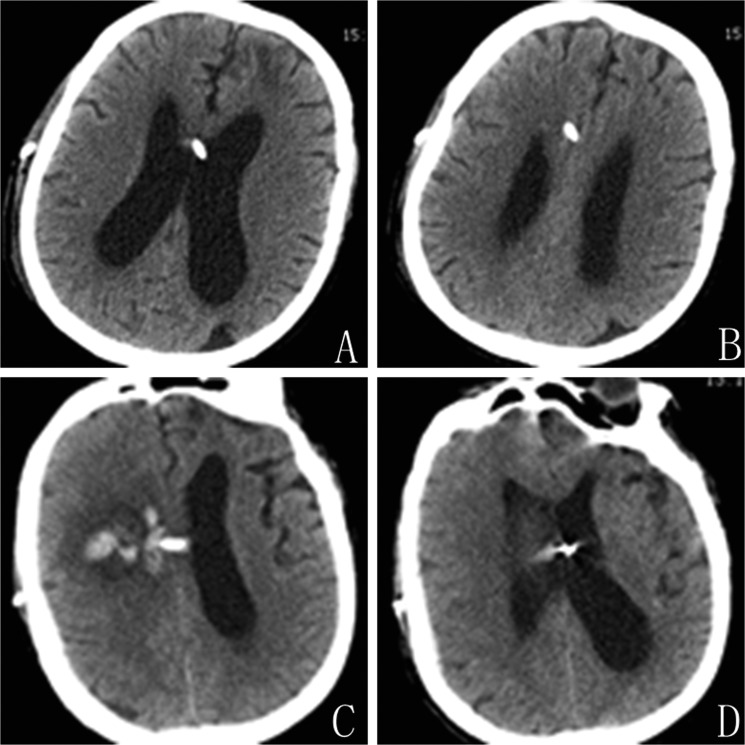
Patient 7. (A and B) Routine CT scans acquired three days after shunt showed shrinking ventricles. (C and D) CT on postoperative day seven showed hemorrhage along the path of ventricular catheter.
